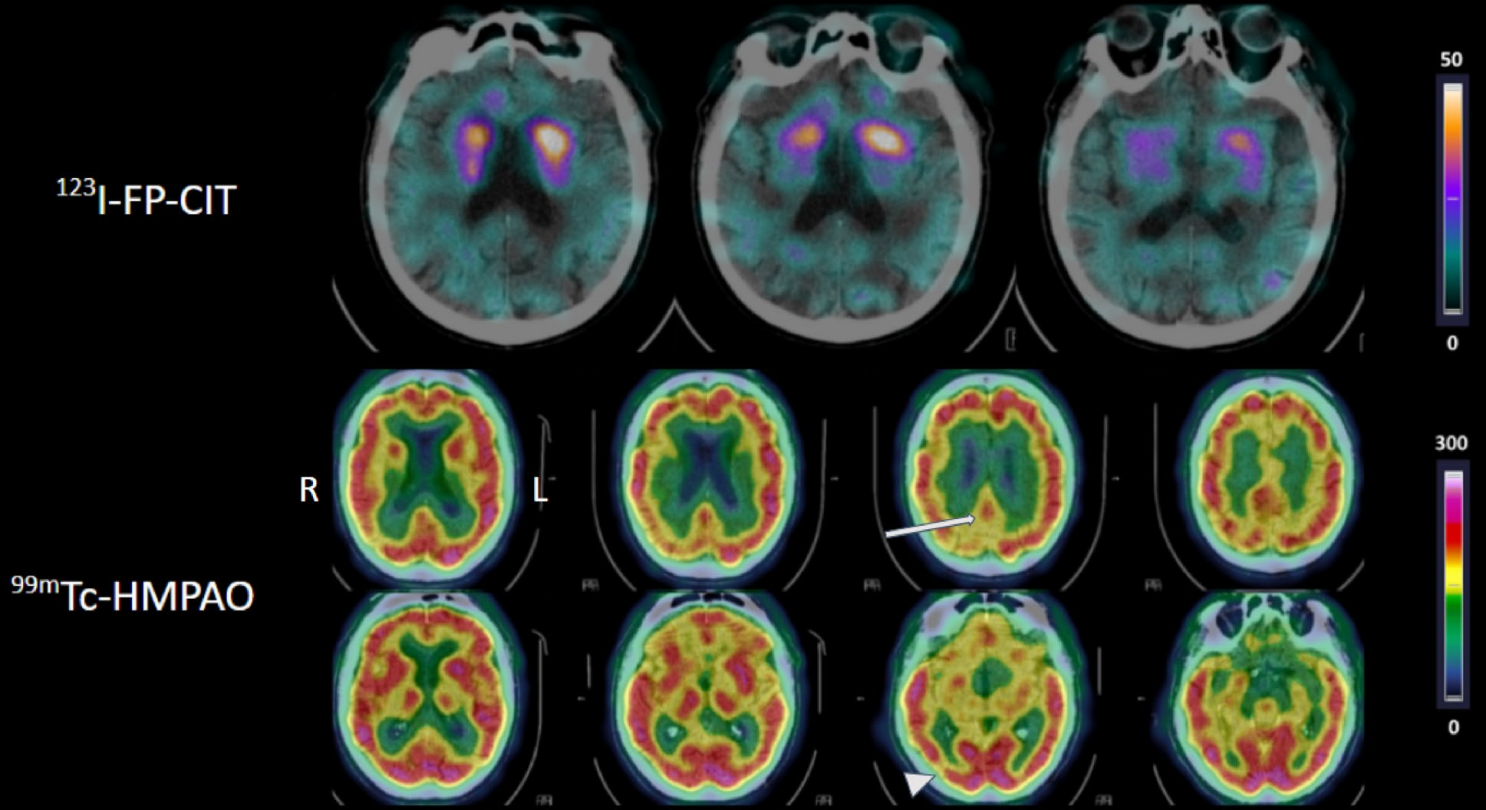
Example of abnormal dual-isotope brain SPECT/CT. The first row displays [^123^I]FP-CIT SPECT/CT axial slices, showing asymmetrical reduced striatal uptake predominantly right. The second and third rows display [^99m^Tc]TcHMPAO SPECT/CT axial slices showing a possible cingulate island sign (arrow) and parieto-occipital hypoperfusion (arrowhead). R: right; L: Left; SPECT: Single Photon Emission Computed Tomography
